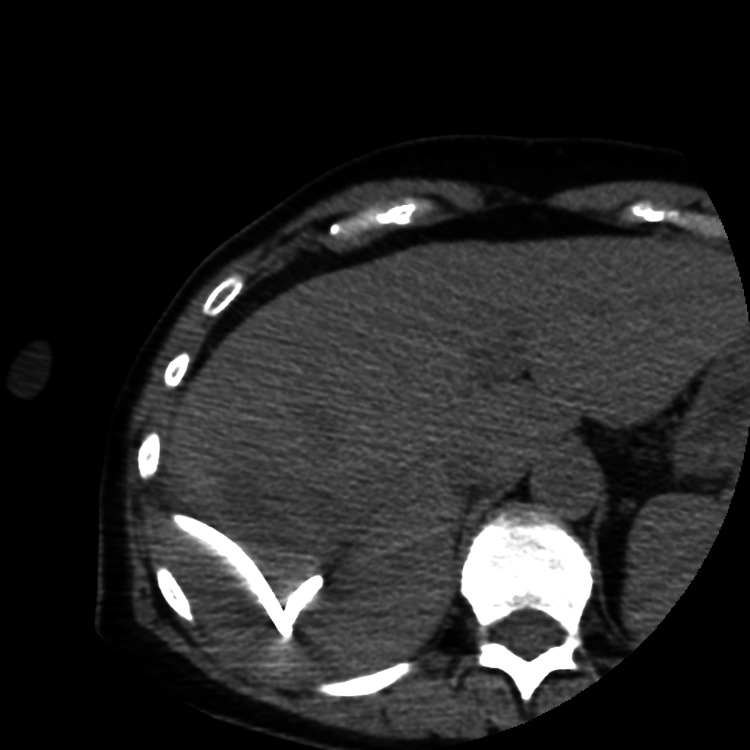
Intra-Procedural Computed Tomography (CT) Image Intra-procedural computed tomography (CT) image demonstrating placement of a 10-French drainage catheter within the hepatic abscess. The catheter is seen coiled in the fluid collection in the right hepatic lobe.
